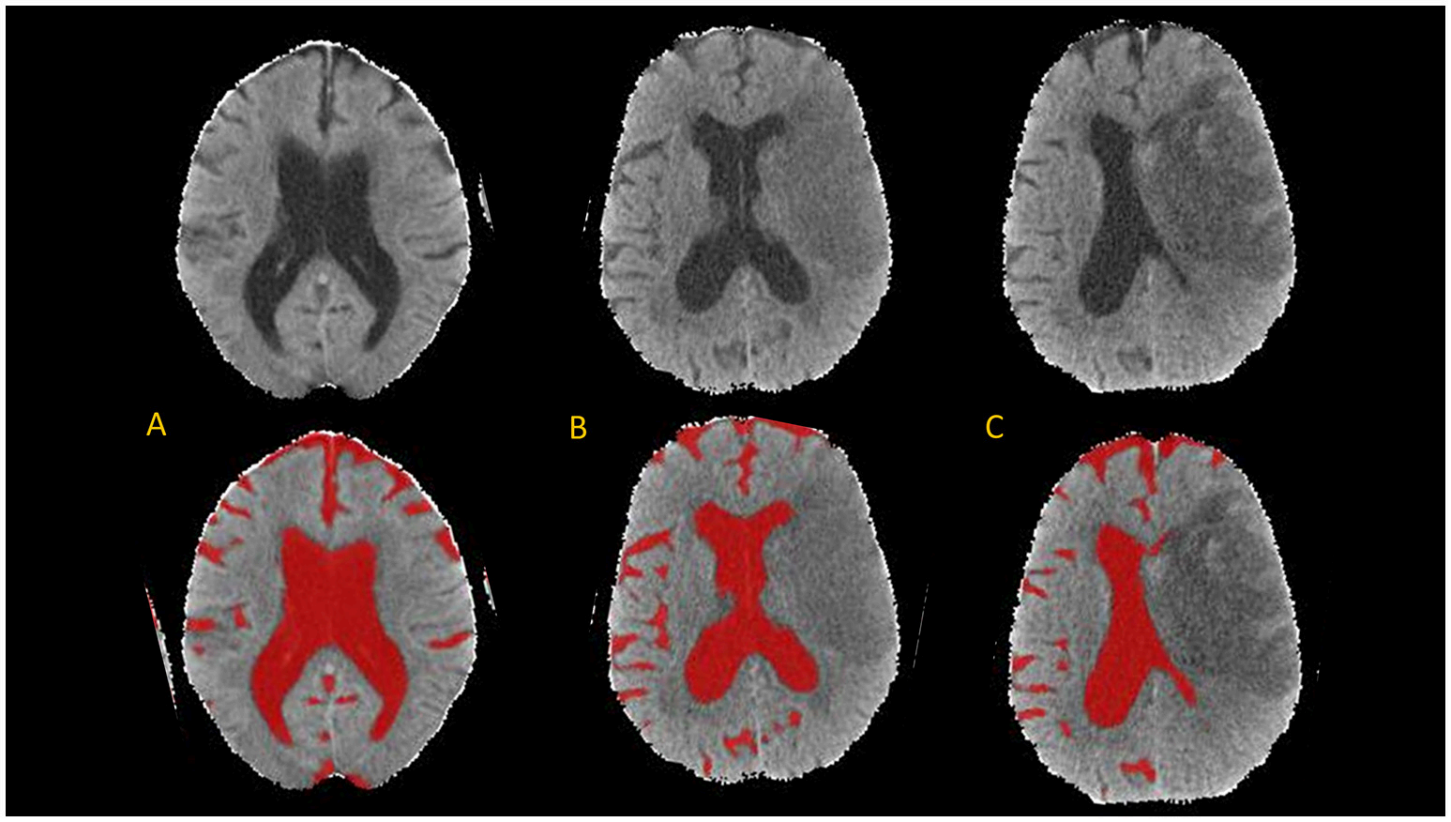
Axial brain slices from head CT (top) and results of CSF segmentation from a 82-year old woman with initial NIHSS of 18. Baseline CT (A) was performed within 1 h of stroke onset (CSF volume 224 ml). First follow-up CT (B) was performed at 20-h (CSF volume 150 ml) and second follow-up CT (C) at 110-h (CSF volume 105 ml).
